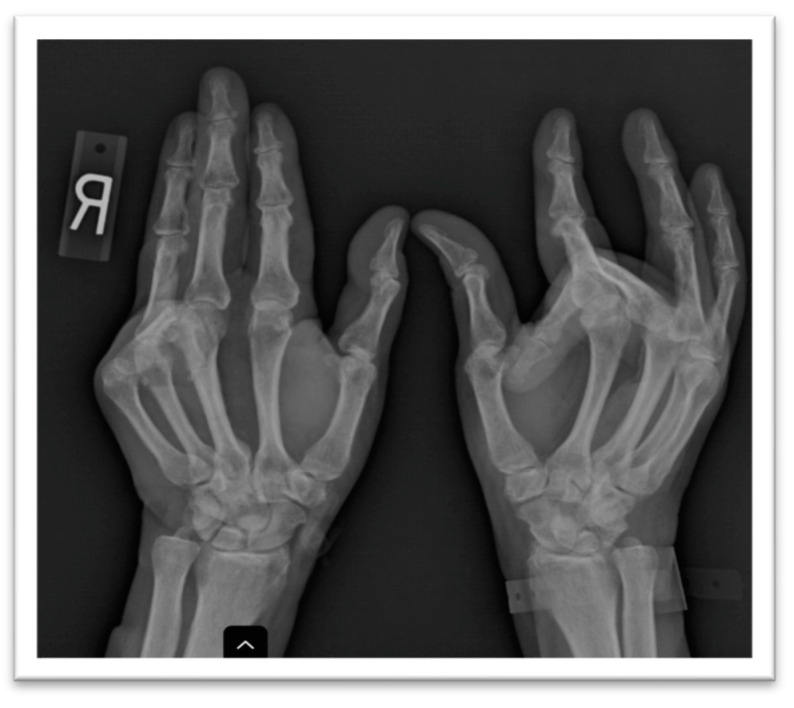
Plain X-ray of hands showing prominent interphalangeal bony productions and subluxations of multiple digits.
